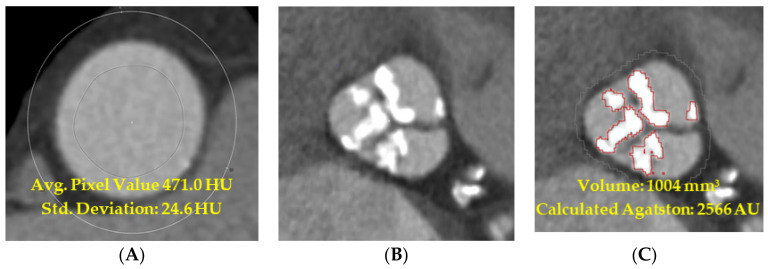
Sample images of a contrast-enhanced computed tomography showing aortic calcification. (A) The dynamic threshold is determined by measuring the attenuation of the ascending aorta adding four times the standard deviation. (B) A visualization of calcification using the new threshold. (C) This new threshold is then used for the semi-automatic detection of aortic calcification. Red-bordered structures = semi-automatic quantifications of a calcified aortic valve.
